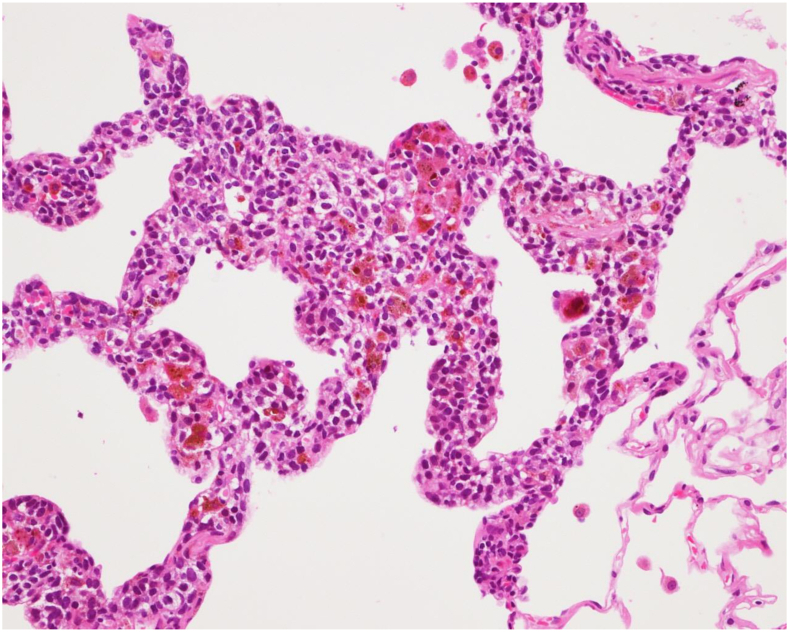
Pulmonary metastases (PMs) presenting with GGN are rare; however, 4.6% of PMs from melanoma can be manifested as GGNs, although solitary cases are limited.^1^ The lepidic growth of the tumor cells led to this radiographic appearance. The reported mean tumor doubling time for PMs from melanoma with GGN appearance is 52 days, which is useful for differentiating them from AIS of 813 days.^1^ In this case, the GGN displayed extremely slow growth, making differentiation from AIS challenging. In patients with history of melanoma, slowly progressive small GGNs with controversial surgical indications should be considered for resection considering the possibility of PMs.
